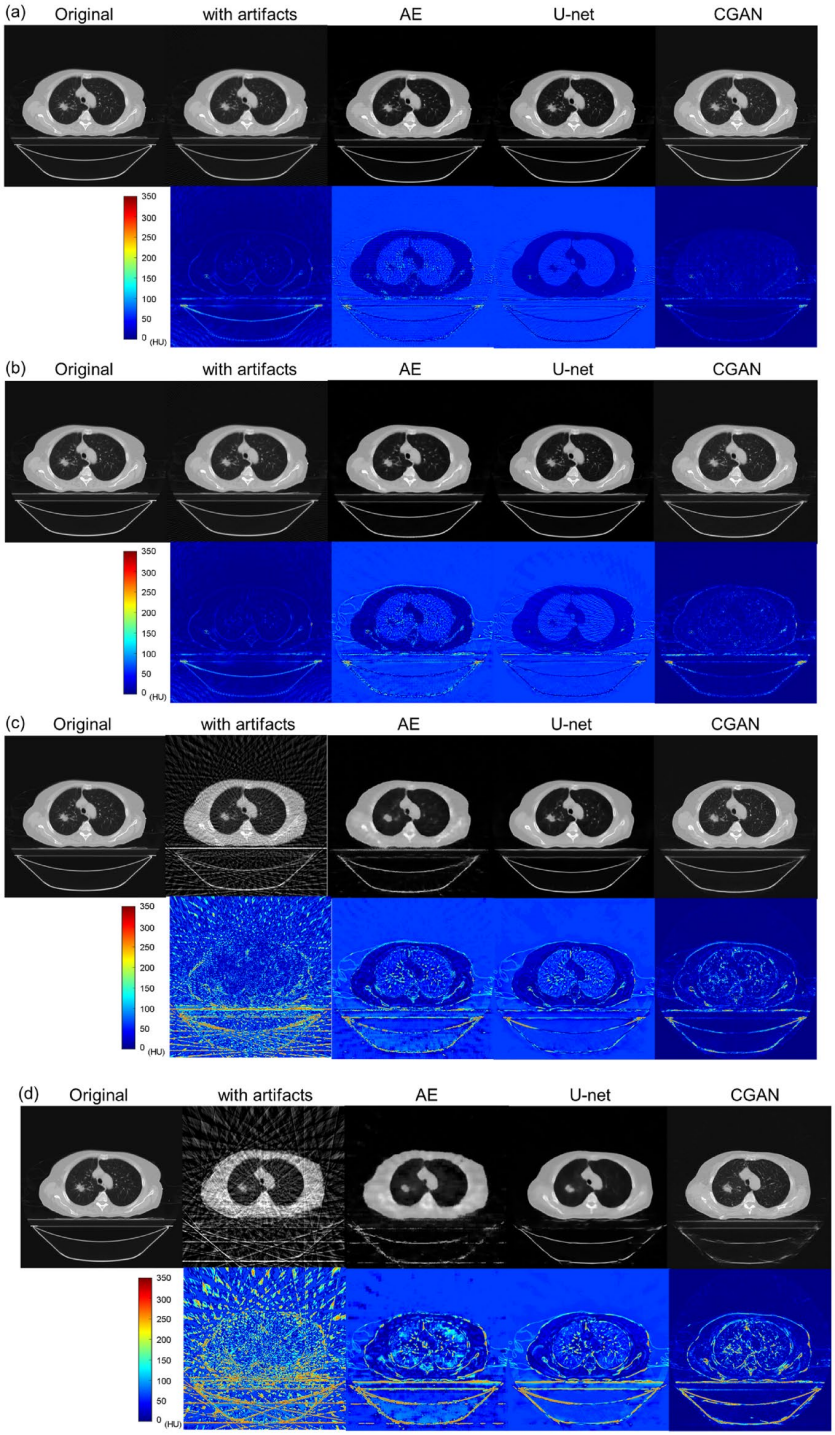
Results of artifact-corrected image in sparse-view CT by AE, U-Net, and CGAN. (a–d) Representative axial slice images with dispersed projection angles of 1°, 2°, 5°, and 10°. Subtraction image, created by subtracting the artifact-corrected image from the original image, is shown at the bottom of each dispersed projection image. All images are shown with the same window width and levels.
